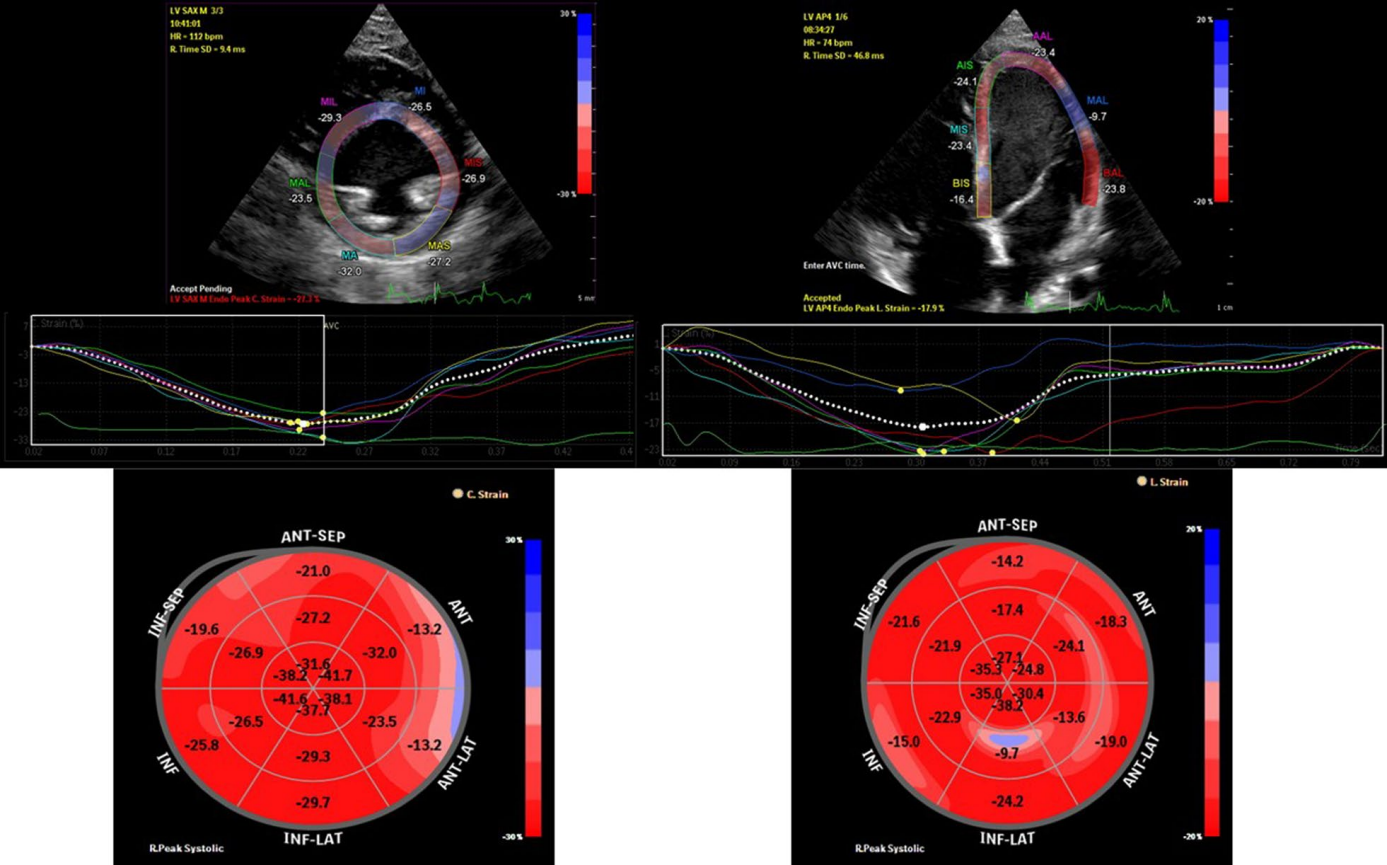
Myocardial strain measurements. The upper panel shows automated tracking of the region of interest at the short-axis mid-ventricular level on the left and the apical four-chamber level on the right. The lower panel shows corresponding 18-segment strain measurements
